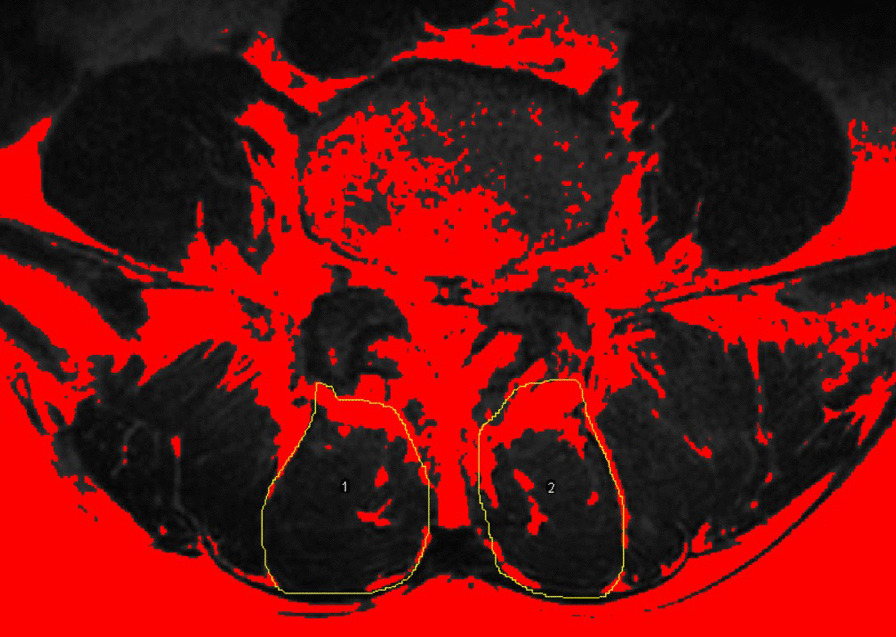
Measurements of paraspinal muscular parameters on axial T2-weighted MRI (a 55-year-old man). Regions of multifidus at L4 level were outlined by yellow lines. Thresholding technique to highlight fatty area (red area)
